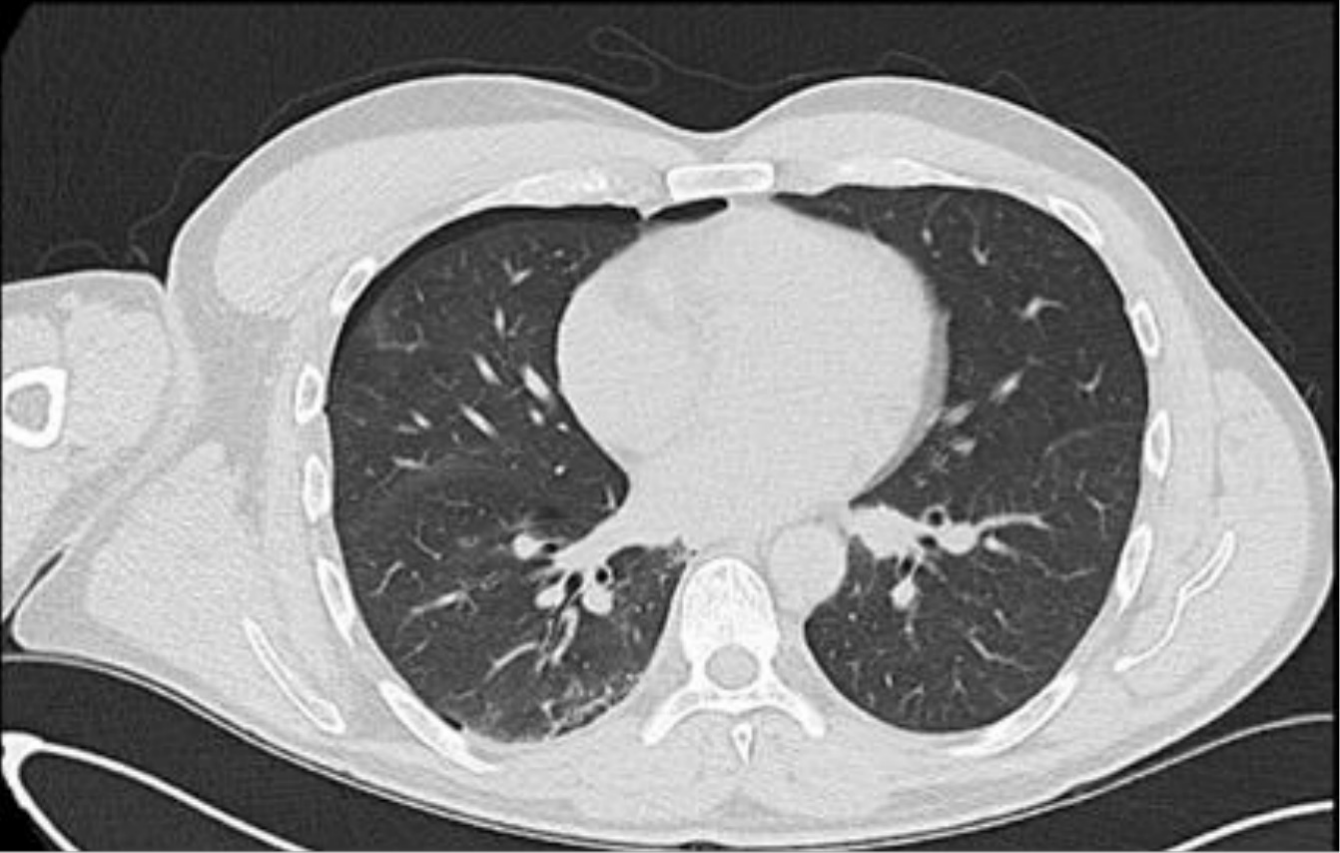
CT Chest, Day of injury, small < 10% pneumothorax on the right side.
